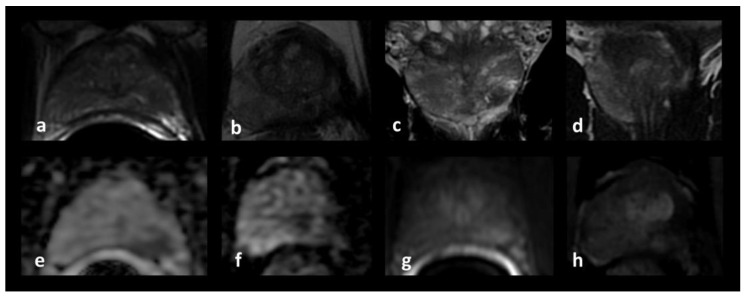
Pre-operative and post-operative multiparametric MRI images. (a): Pre-operative axial T2-weighted image. (b): Post-operative axial T2-weighted image. (c): Pre-operative coronal T2-weighted image. (d): Post-operative coronal T2-weighted image. (e): Pre-operative axial ADC (apparent diffusion coefficient) map image. (f): Post-operative axial ADC map image. (g): Pre-operative axial perfusion image. (h): Post-operative axial perfusion image.
